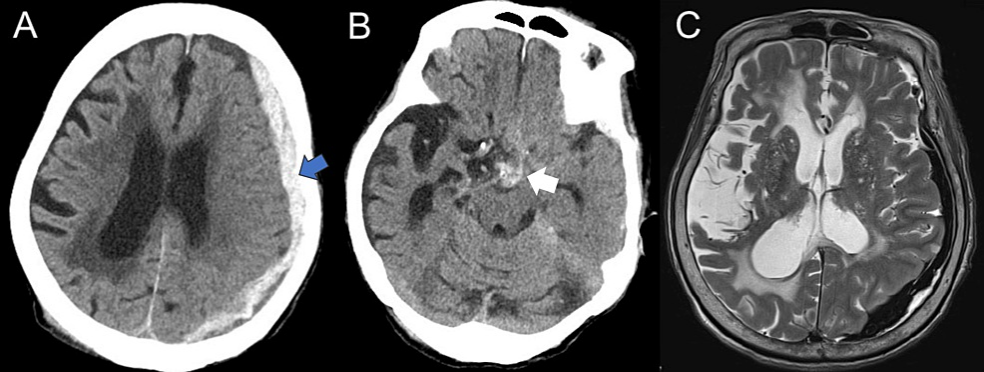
Initial computed tomography imaging and magnetic resonance imaging (A,B) Initial computed tomography (CT) images showing left hemispheric acute subdural hematoma (ASDH) (blue arrow) and mild traumatic subarachnoid hemorrhage in the interpeduncular cistern (white arrow). Magnetic resonance imaging (MRI) showing the same lesions, with hypointensity in the axial T2-weighted image (C). Additionally, MRI and CT showed hydrocephalus ex vacuo and the right predominant periventricular cerebrospinal fluid (CSF) leaks. No contusion or diffuse axis injuries were observed.
